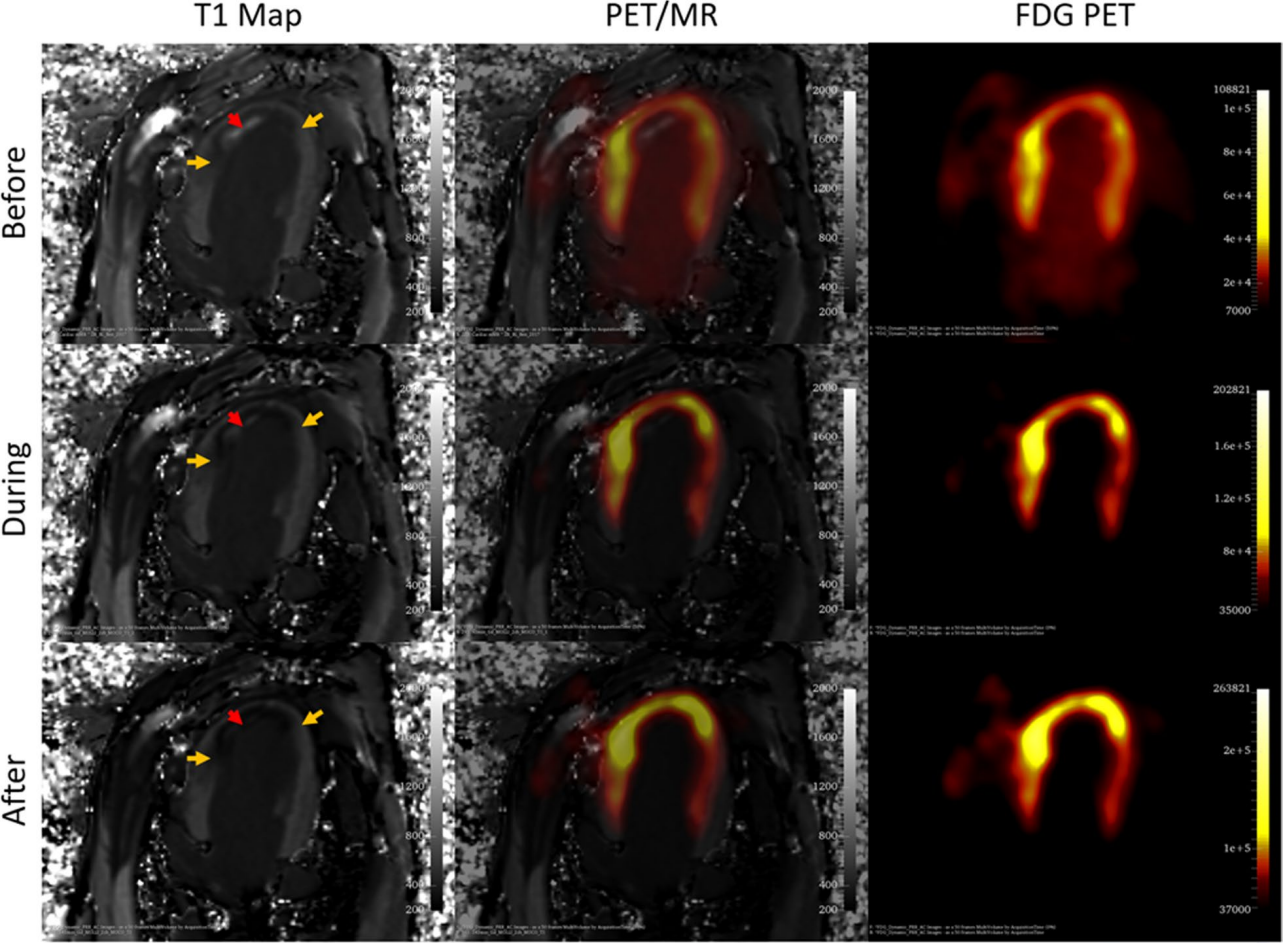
Images were taken before suppression, at 40 min, during suppression, at 90 min and after suppression, at 150 min. FDG-PET images are 3 min frames finishing at the indicated time. Red arrows show the location of infarcted obstructed tissue, while orange arrows show the edge of infarcted not obstructed tissue. Note that the intensity range is changed to account for infusion of activity
